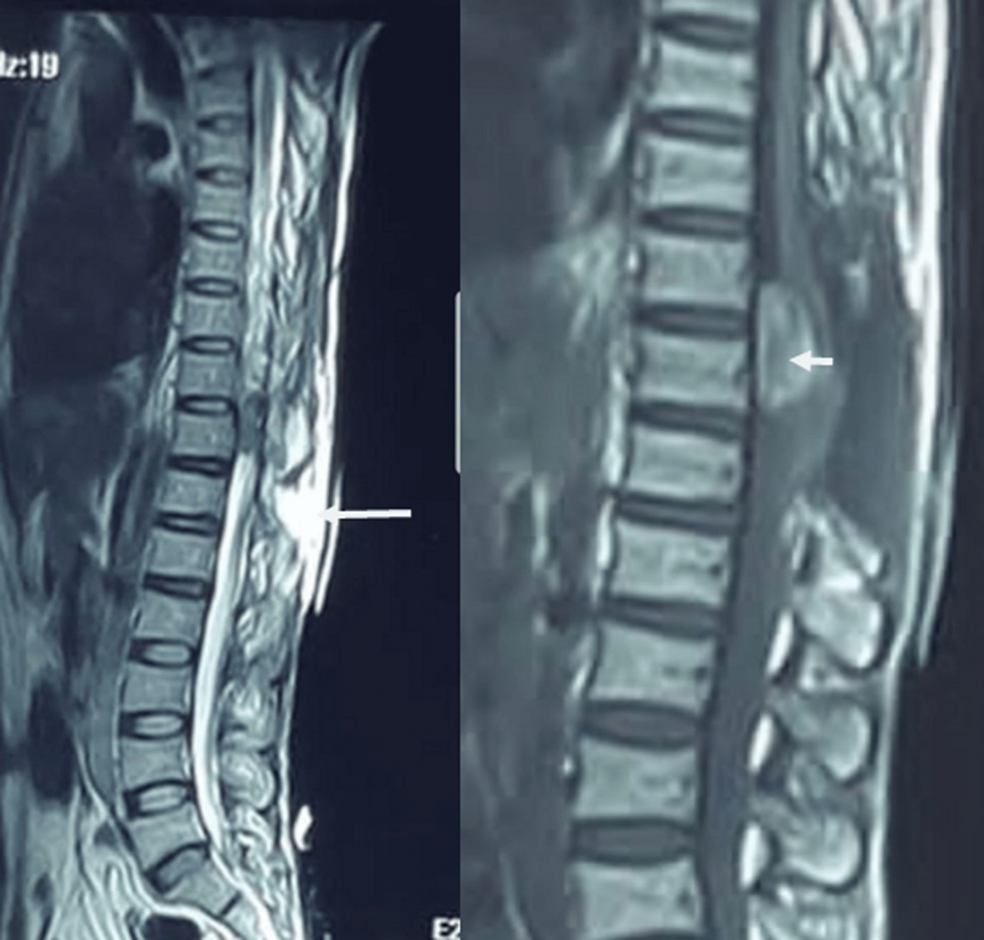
Magnetic resonance imaging image on the left shows a mass at D9/D10 levels. The image on the right shows a yellowish, exophytic mass present ventral to the cord, pushing it dorsally.
